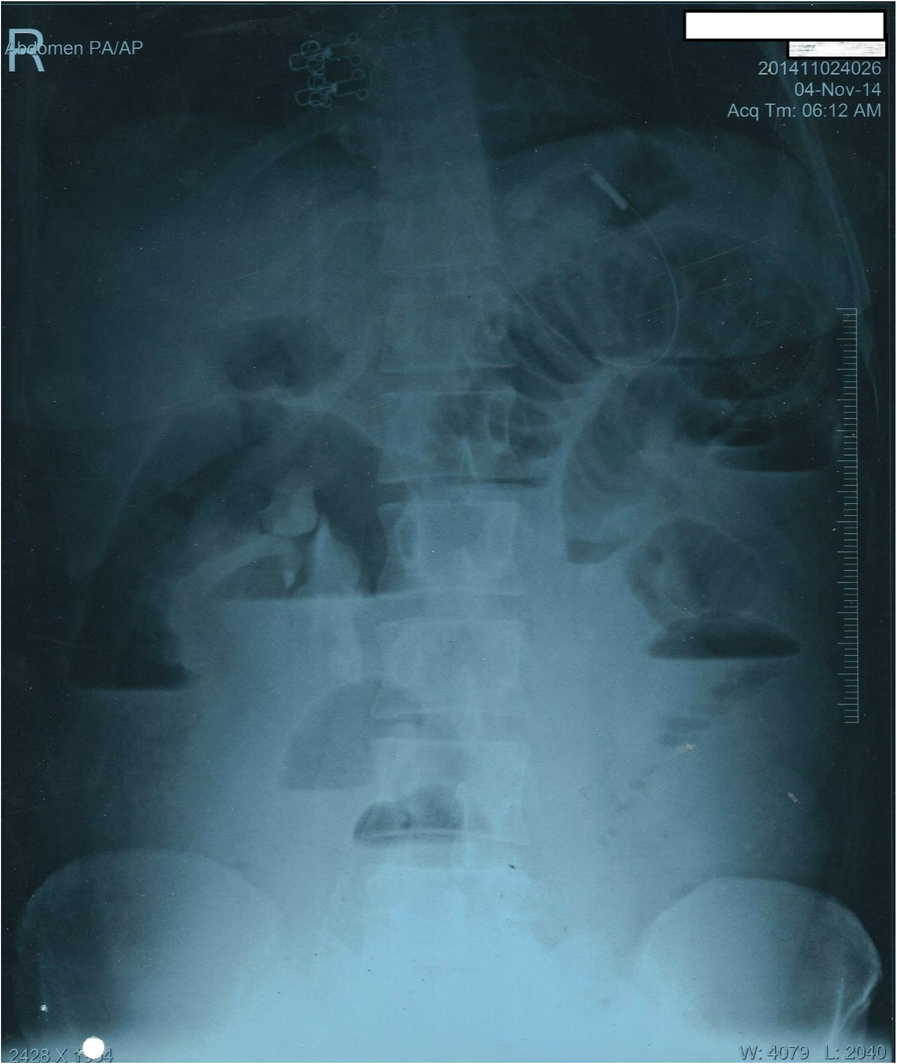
Abdominal radiograph erect showing, multiple air-fluid levels
